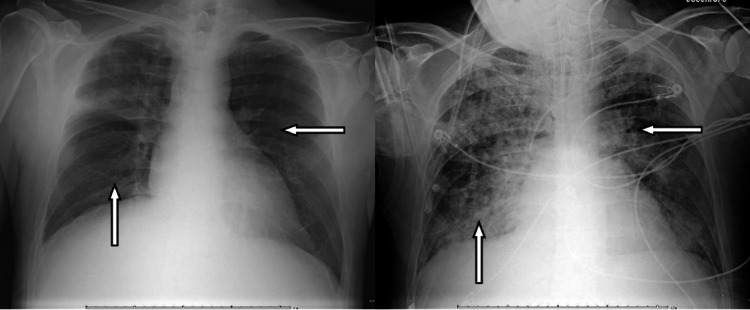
Changes seen in the chest X-ray between day 1 and day 5. The left image shows clear lungs, whereas the right image shows diffuse interstitial and alveolar infiltrates.
